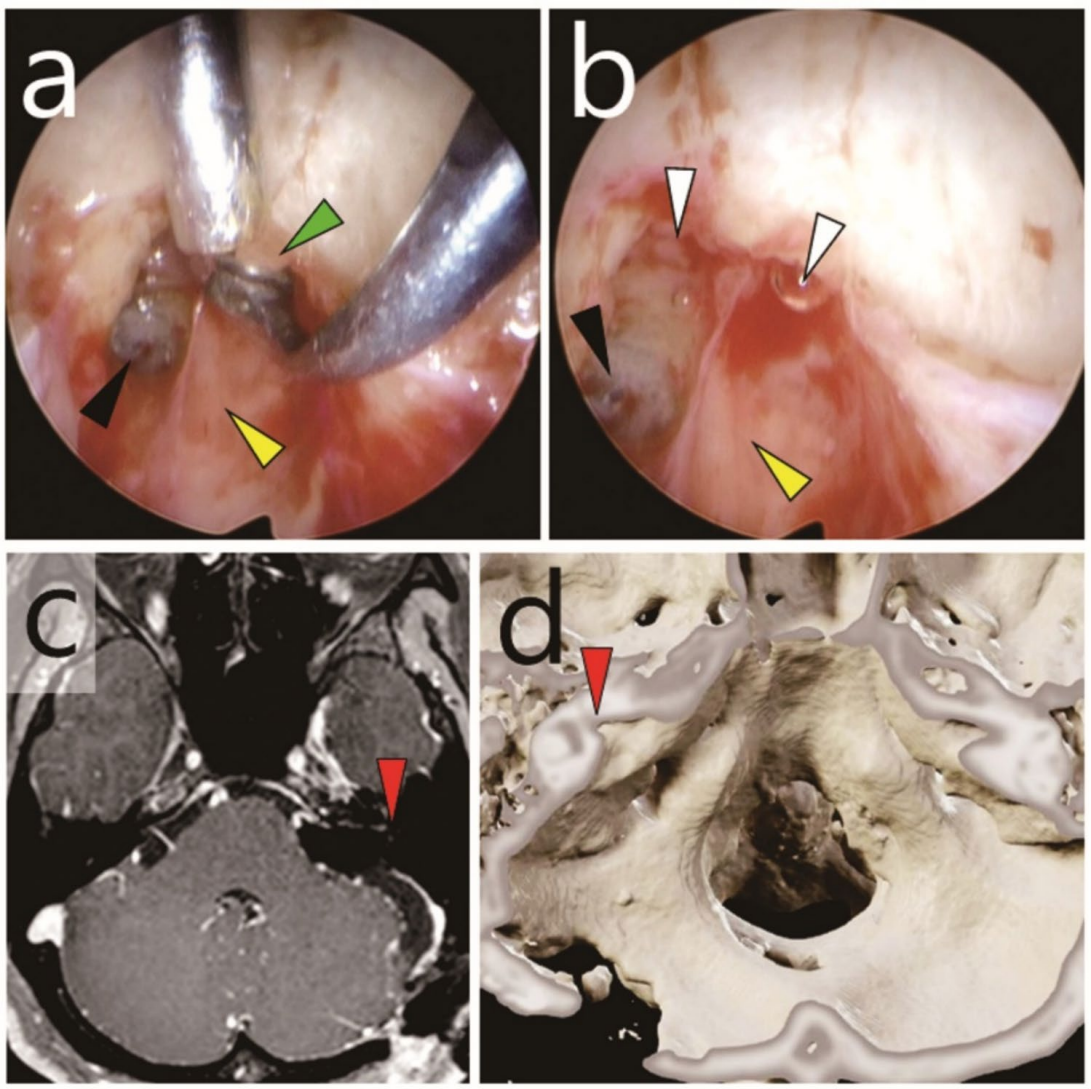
Case 1: Recurrent vestibular schwannoma originating from residual intracanalicular disease. a, b Intraoperative endoscopic photographs. a Resection of the residual tumour (green arrowhead) in the superior vestibular area under 70-degree endoscopic visualisation. Black arrowhead, cochlear area; yellow arrowhead, facial nerve. b Observation of the fundus of the internal auditory canal (FIAC) using a 70-degree endoscope. White arrowheads, vestibular areas; black arrowhead, cochlear area; yellow arrowhead, facial nerve. c Post-salvage surgery gadolinium-enhanced T1-weighted MRI. Red arrowhead, FIAC (d) Post-salvage surgery 3D CT. Red arrowhead, FIAC
